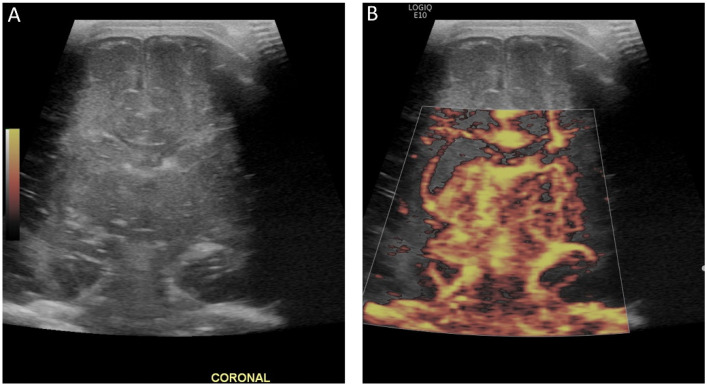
(A) Grayscale and (B) color microvascular imaging ultrasound a 13 day old, former 33 week 5 day infant with congenital heart disease and hypoxic respiratory failure on extracorporeal membrane oxygenation with seizures. Elevated flow is seen within the deep gray matter. This may reflect perfusion alterations in the setting of seizure, dysfunctional autoregulation, and/or evolving injury.
